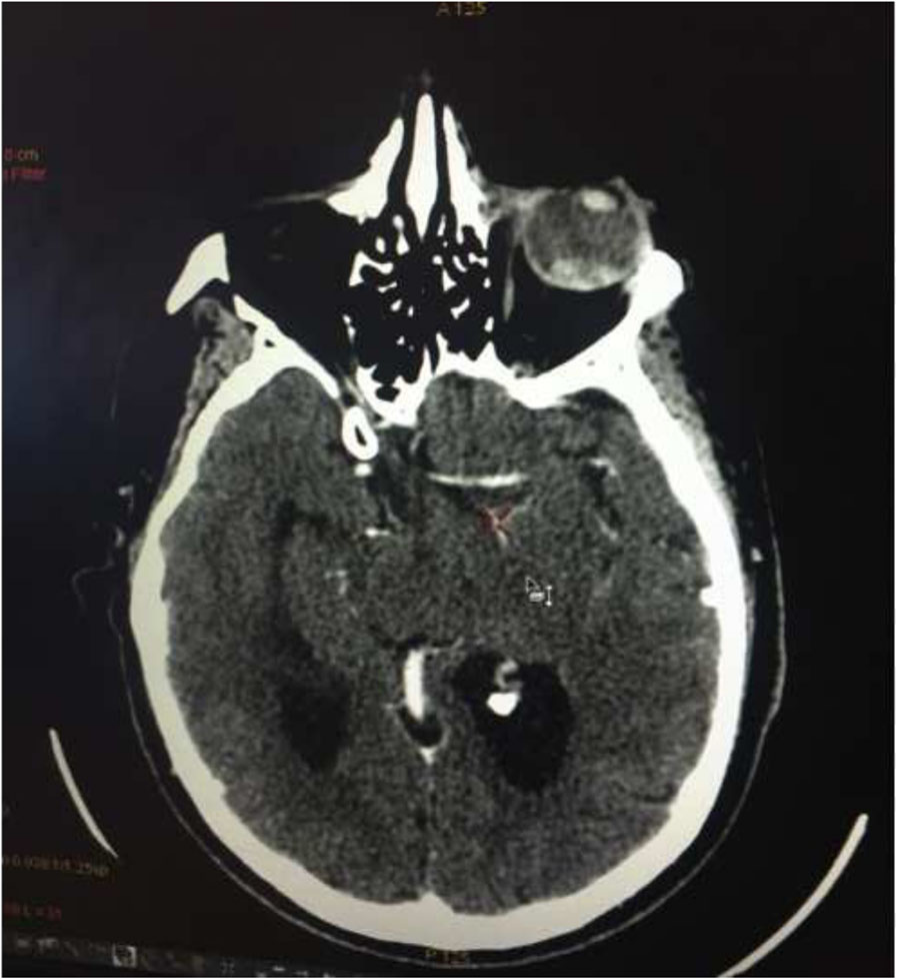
Cross-sectional computed tomographic scan showing a retinal mass
